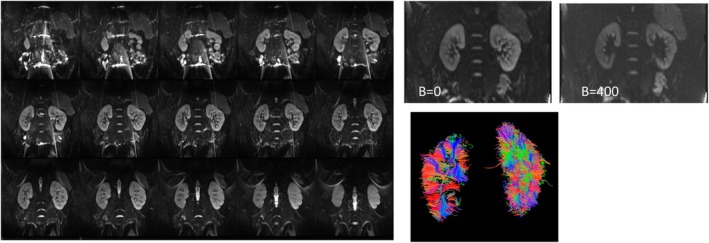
Representative DTI of an 18‐year‐old control female subject showing the kidney slices coverage, B0 image, B400 image of a 20‐direction DTI acquired on a Siemens 3 T MRI. An inset shows the post‐processed DTI tracks.
